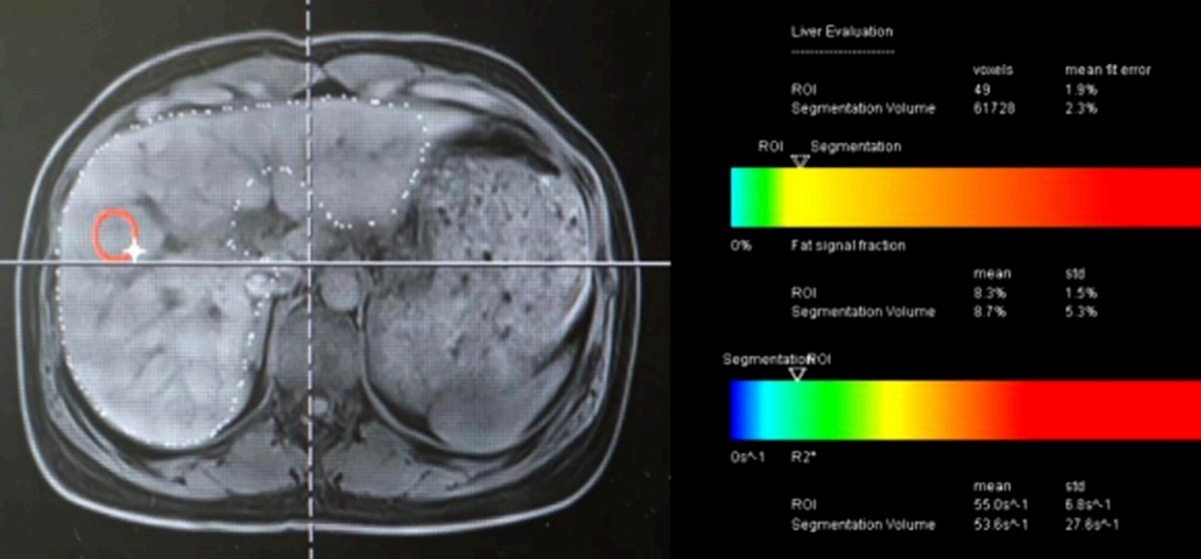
MRI-PDFF measurement on a volunteer with mild hepatic steatosis (MRI-PDFF = 8.3%).
